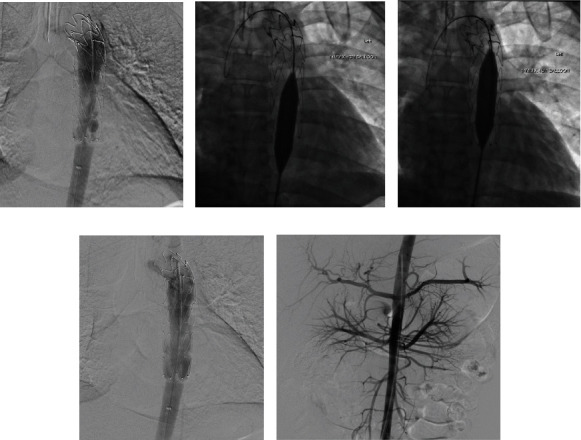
(a) Fluoroscopy post intra-arterial and systemic TPA. (b, c) The stenotic segment in the distal aspect of the stent was treated with 2 overlapping angioplasty deployments using a 14 mm × 4 cm balloon and following of a 16 mm × 4 cm balloon. (d) The final hand-injected aortic run through a 5F straight flush catheter shows mild improvement of the in-stent stenosis. (e) Abdominal aortogram confirming renal parenchymal hypoperfusion in accordance with prior CT findings and complete anuria.
